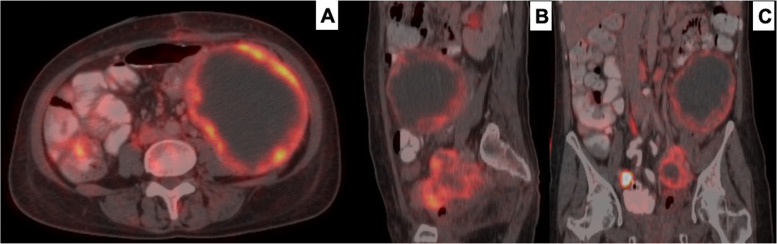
Whole body 18F-fluorodeoxyglucose (FDG) positron emission tomography (PET)-contrast-enhanced computed tomography (CECT) of the patient 6 weeks after ileal resection for anastomotic metastases showed multiple, large, necrotic omental and serosal lesions in abdomen and pelvis regions, and pelvic lymph nodes showing central necrosis and FDG avidity in the walls, largest lesion measuring 12.5 × 11.7 × 11.5 cm (SUVmax 13.8) in left lumbar region in A axial, B sagittal, and C coronal views
